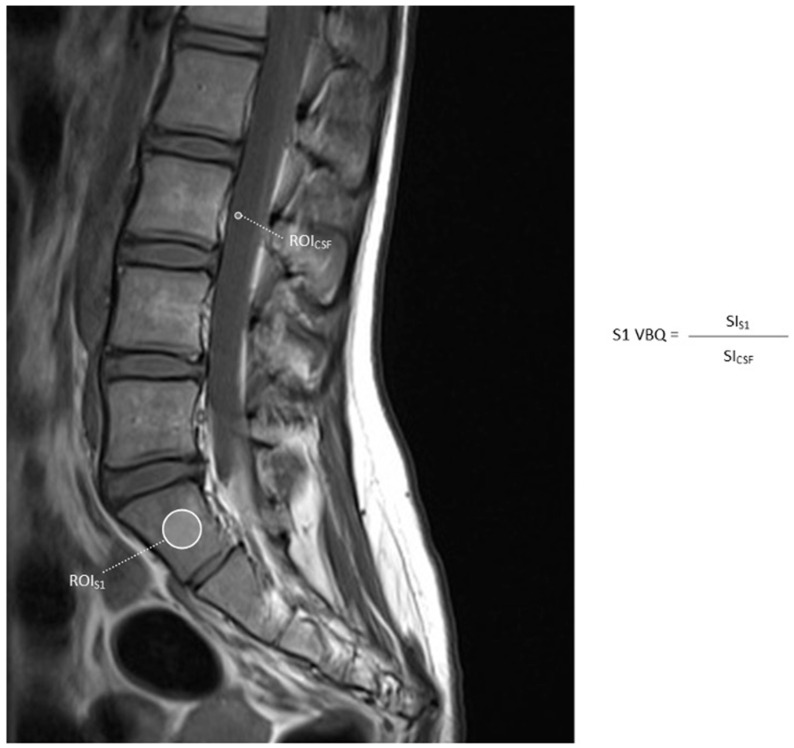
Non-contrast-enhanced T1-weighted MRI of the lumbosacral spine showing ROI used to calculate S1-VBQ score.
